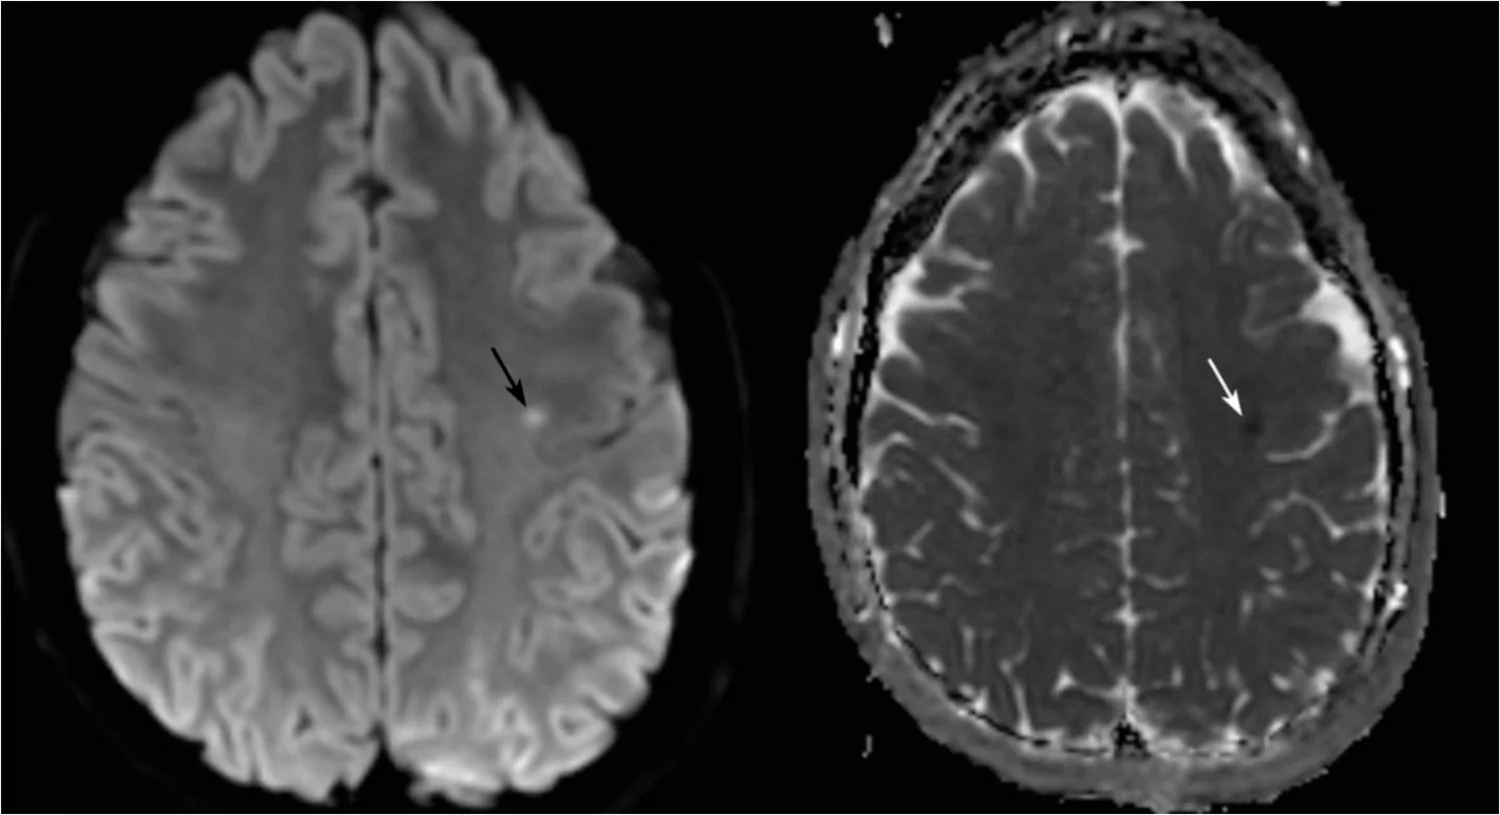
MRI brain. Left: Diffusion-weighted imaging (DWI) shows a punctate focus of increased signal in the subcortical white matter of the left parietal lobe (black arrow). Right: The apparent diffusion coefficient (ADC) map reveals a corresponding punctate hypo-intense signal in the same region indicative of a true acute ischemic infarct. Original radiology images from the patient, reviewed and annotated by M.T
